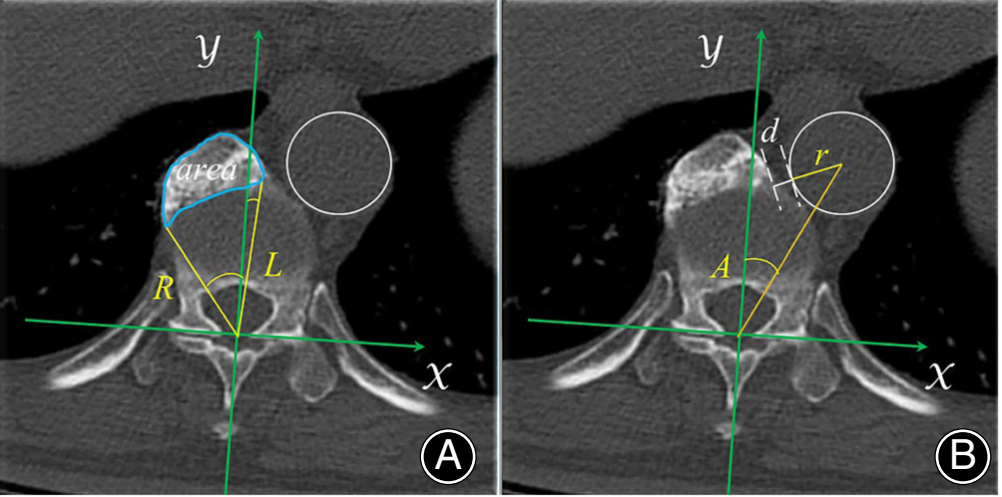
The parameters measured on an axial CT scan. (A) area: the area of osteophyte at the selected scan. R & L: angle separately formed by the y‐axis and a line connecting the origin and the right and left margin of osteophyte. (B) A: angle formed by the y‐axis and a line connecting the origin and the center to the aorta. d: the distance between the aorta and the nearest margin of the osteophyte. r, the radius of the aorta.
